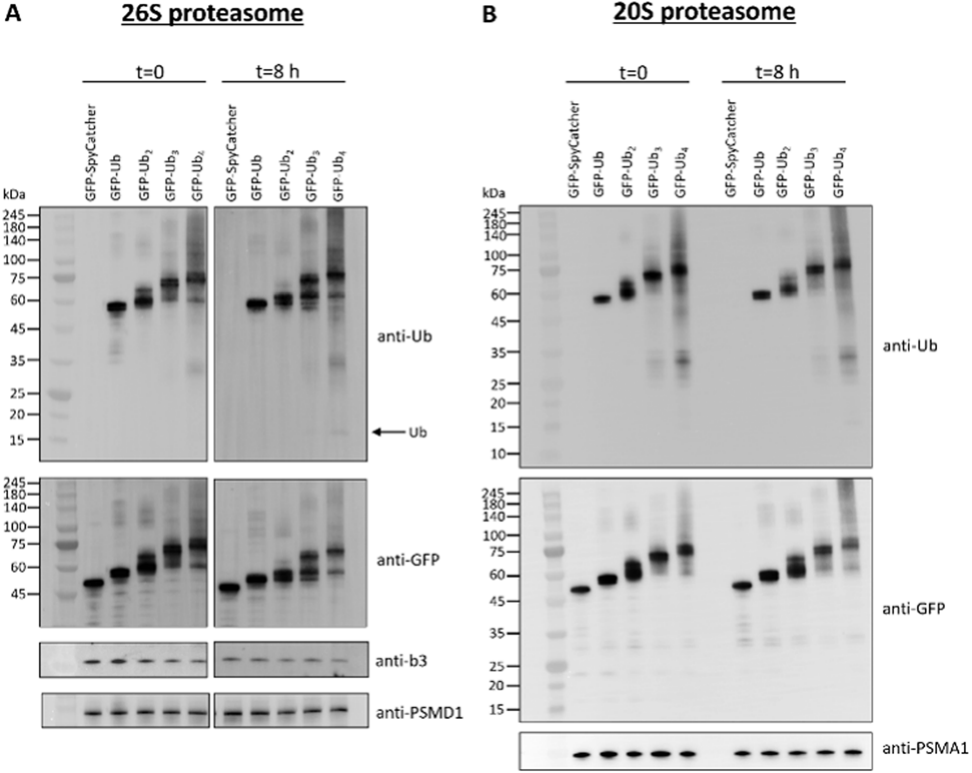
Proteasomal degradation of eGFP-Ub conjugates. (A) Degradation by the 26S proteasome. (B) Degradation by the 20S proteasome. Antibodies against PSMD1 (19S proteasome non-ATPase regulatory subunit 1), PSMA1 and b3 (20S proteasome subunit alpha type-1 and beta type-3 subunit, respectively) were used as proteasome loading controls.
